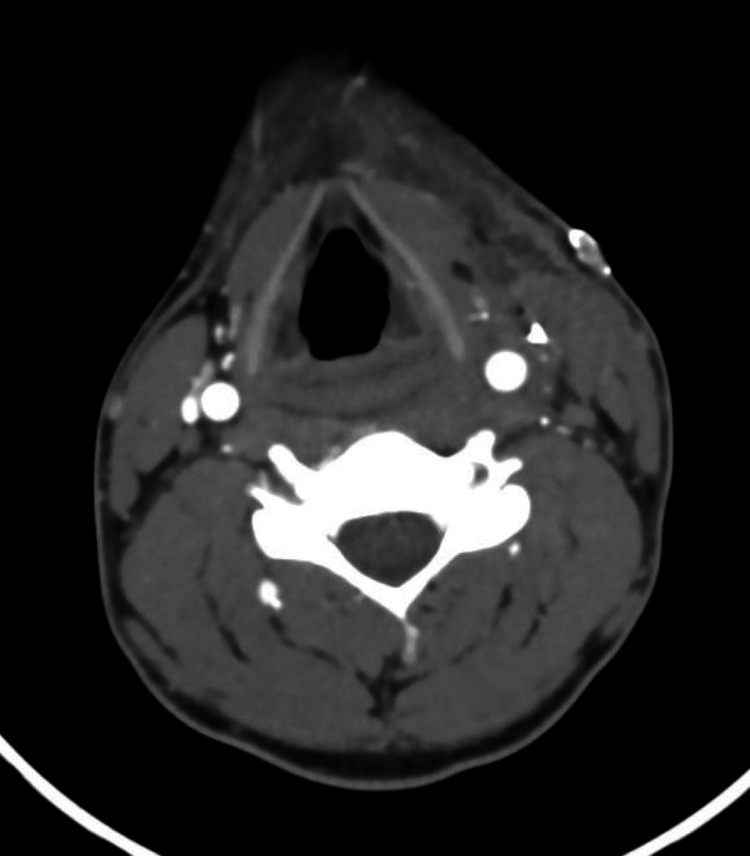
Postoperative CT (axial plane). Postoperative changes with no obvious contrast extravasation, dissection, or wall irregularities were observed.
